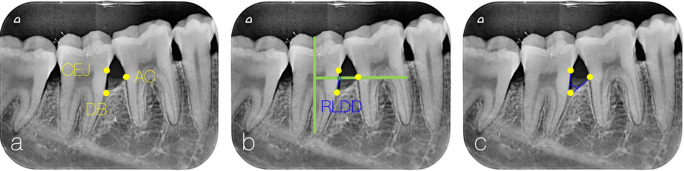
Intra-osseous defect radiographic measurements. a Identifying reference points: cementoenamel junction (CEJ), defect base (DB) and alveolar crest (AC). b Identifying reference lines (in green): vertical line corresponding to long axis and horizontal perpendicular line passing through AC, and identifying radiographic linear defect depth (RLDD) in blue. c Radiographic angle connecting CEJ, DB and the lateral border of the defect
